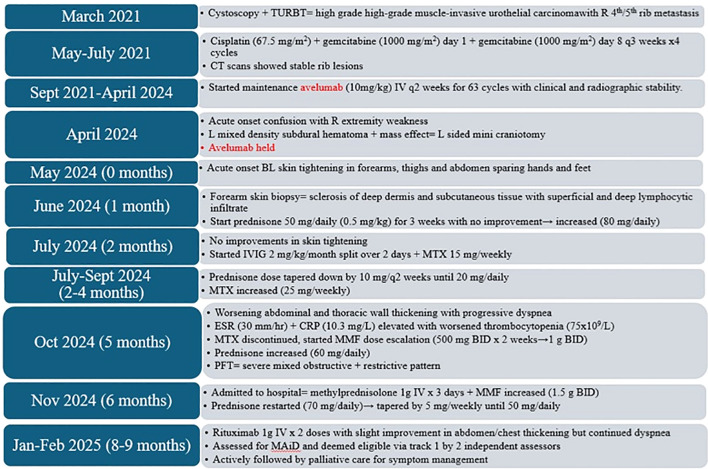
Treatment course for case 1. Timeline in months from onset of eosinophilic fasciitis. TURBT, trans-urethral resection of bladder tumour; R, right; L, left; q, every; CT, computer tomography; BL, bilateral; IVIG, intravenous immunoglobulin; MTX, methotrexate; ESR, erythrocyte sedimentation rate; CRP, C-reactive protein: MMF, mycophenolate; BID, twice a day; PFT, pulmonary function test; MAiD, medical assistance in dying.
